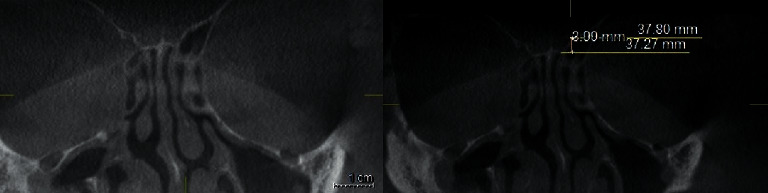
Coronal plan of CBCT demonstrating type 1 Keros classification on the left side.
